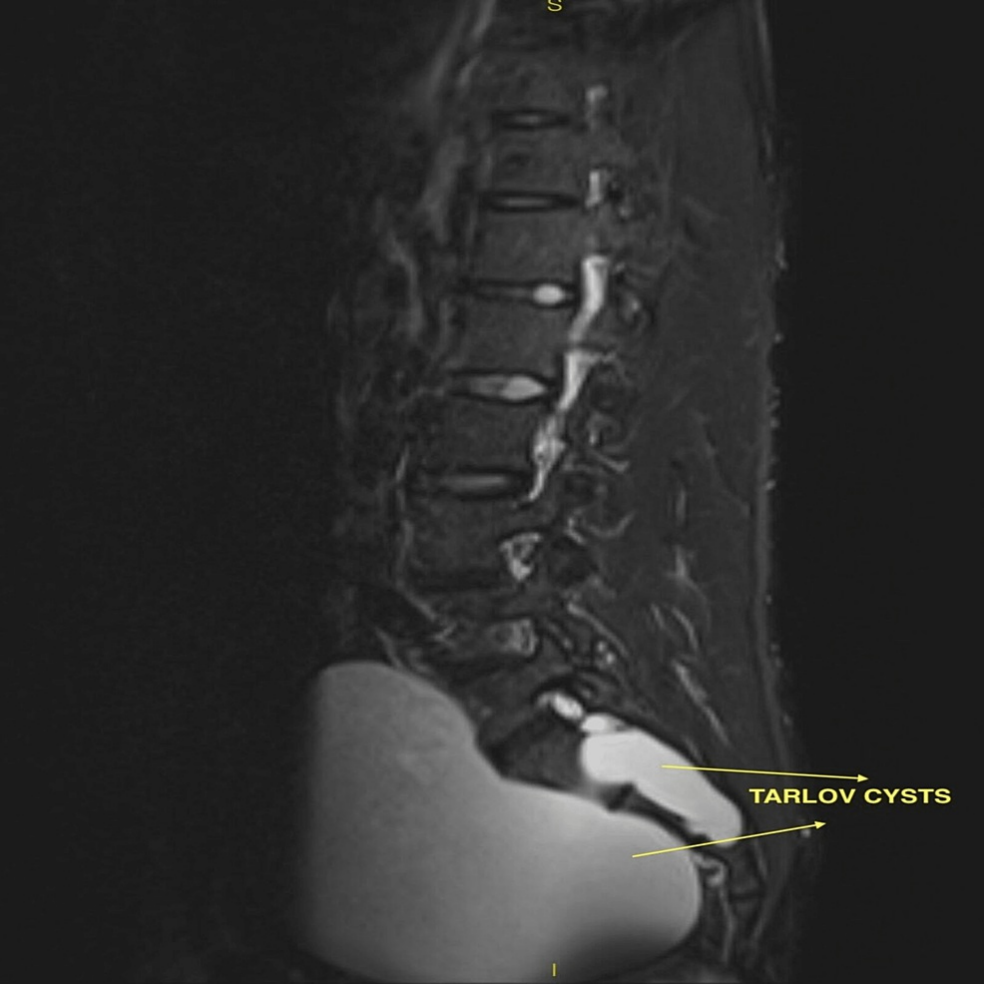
T2 MRI sagittal image showing giant Tarlov cysts
